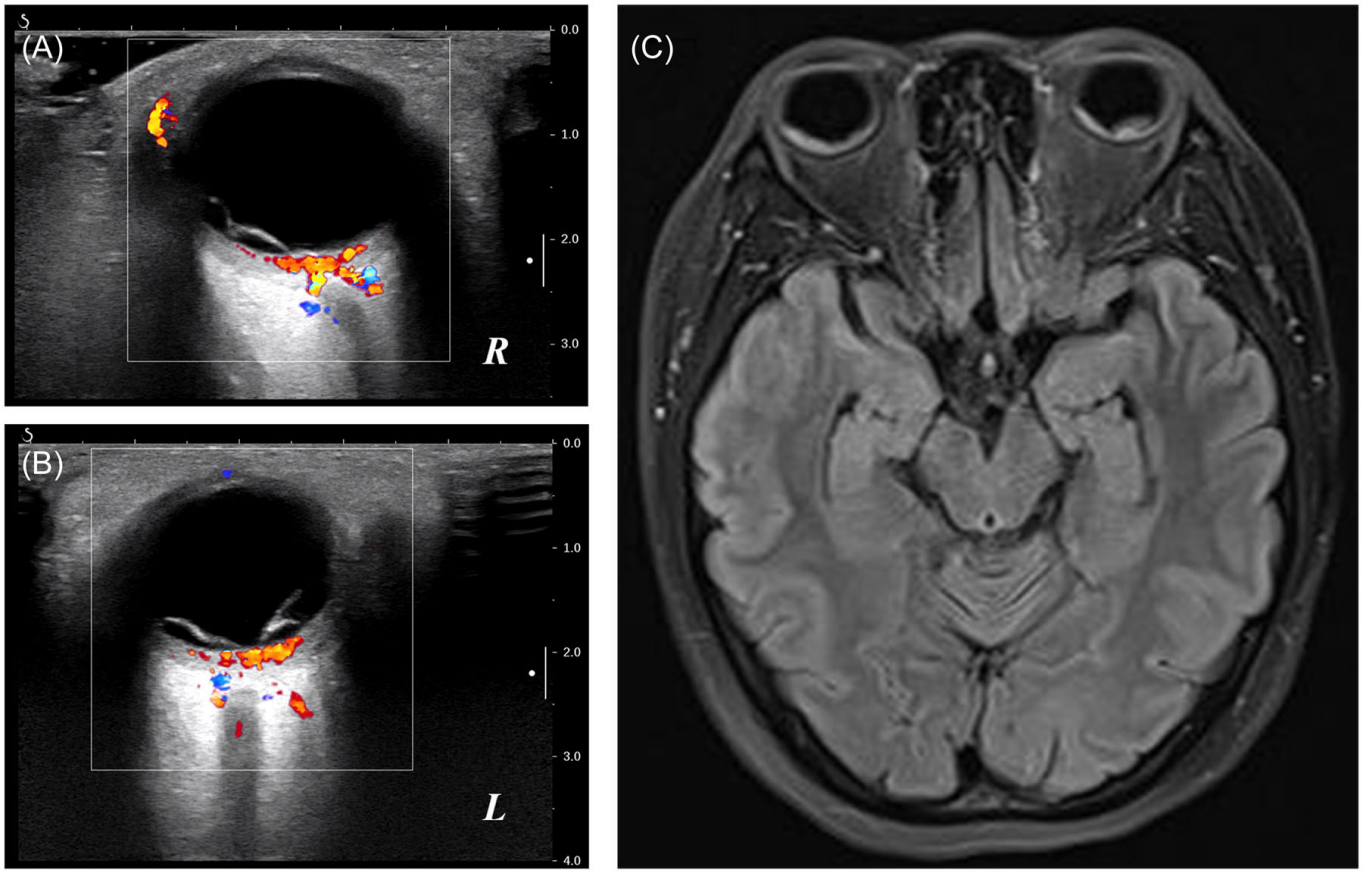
B‐scan ultrasonography findings highlighting serous retinal detachment and thickened choroid in the right eye (A) and the left eye (B). Brain MRI images (C) demonstrating bilateral retinal detachment. MRI, magnetic resonance imaging.
